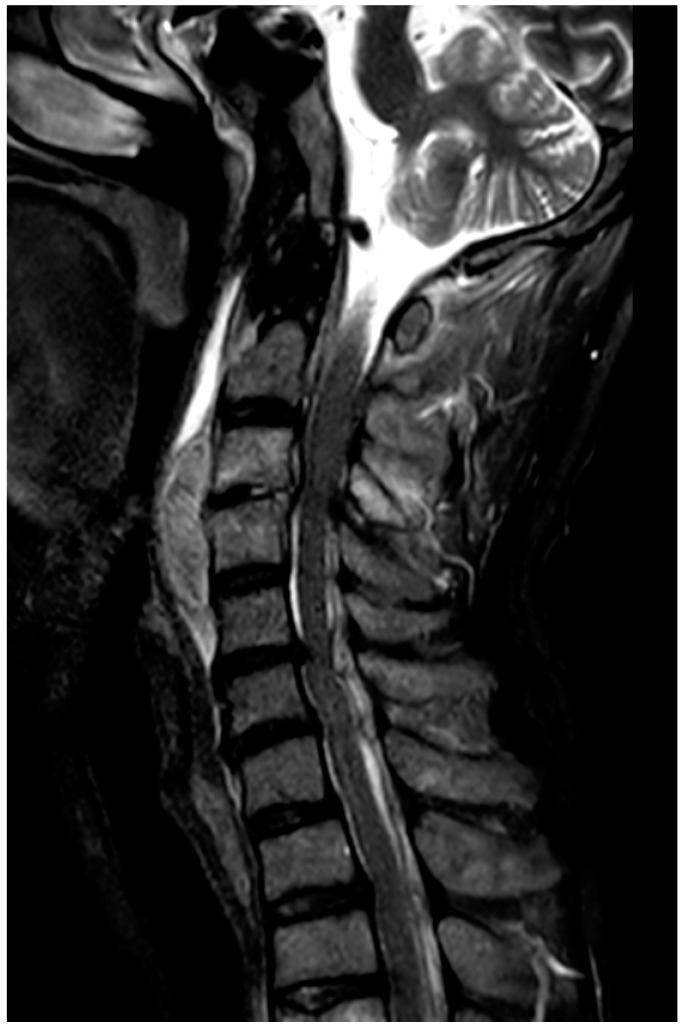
MRI T2 STIR. Mid sagittal STIR MRI on admission (day 0) showing a dorsal epidural lesion at C3–C5 compressing the spinal cord; high signal with rim enhancement consistent with secondary infection of a post-traumatic hematoma.
